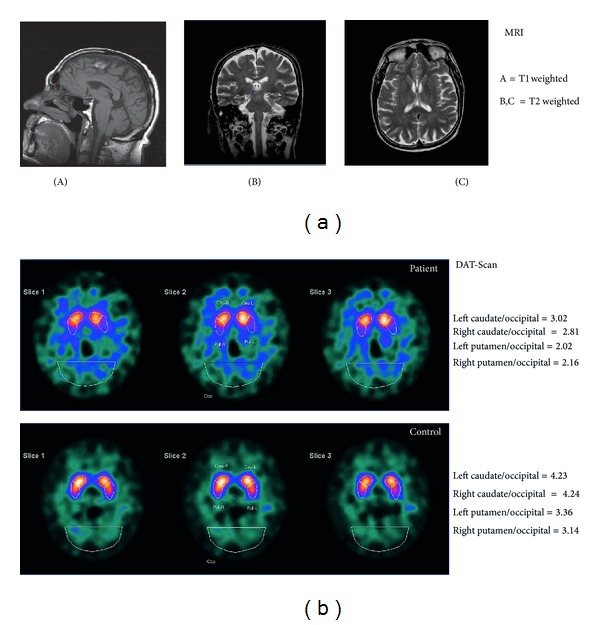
(a) Sagittal (A), coronal (B), and axial (C) magnetic resonance imaging (MRI) scans of the patient at the age of 77 years. (b) ^¹²³^FP-CIT SPECT imaging of the patient at the age of 78 years and an age- and sex-matched normal control.
